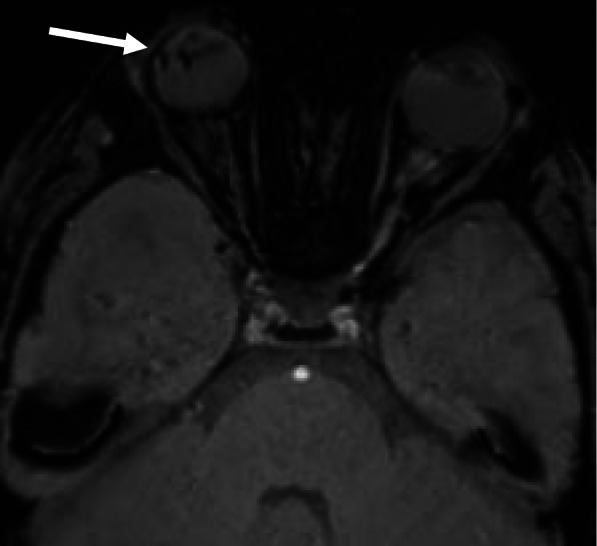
MRI brain axial susceptibility-weighted images demonstrate curvilinear susceptibility artefact (arrow) associated with the right lens, suggestive of retinal detachment
